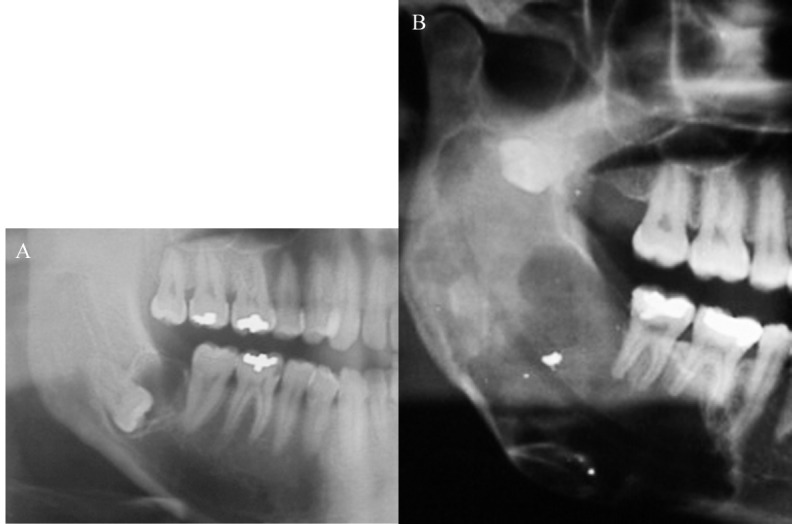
Orthopantomografic views. A) Orthopantomografic view of the case 2. B) Orthopantomografic view of the case 4.
